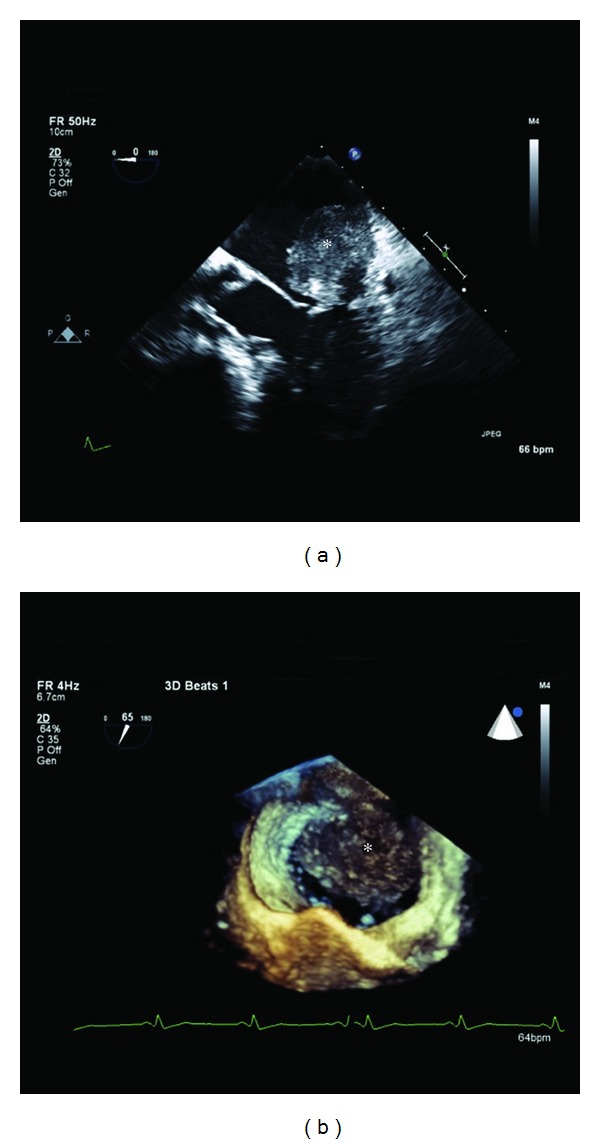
Two-dimensional (a) and magnified three-dimensional (b) transesophageal echocardiogram (TEE) of the left atrium (LA), mitral valve, and left ventricle. A 4.4 × 3.5 cm mass (asterisk) is seen attached to the posterolateral aspect of the LA.
